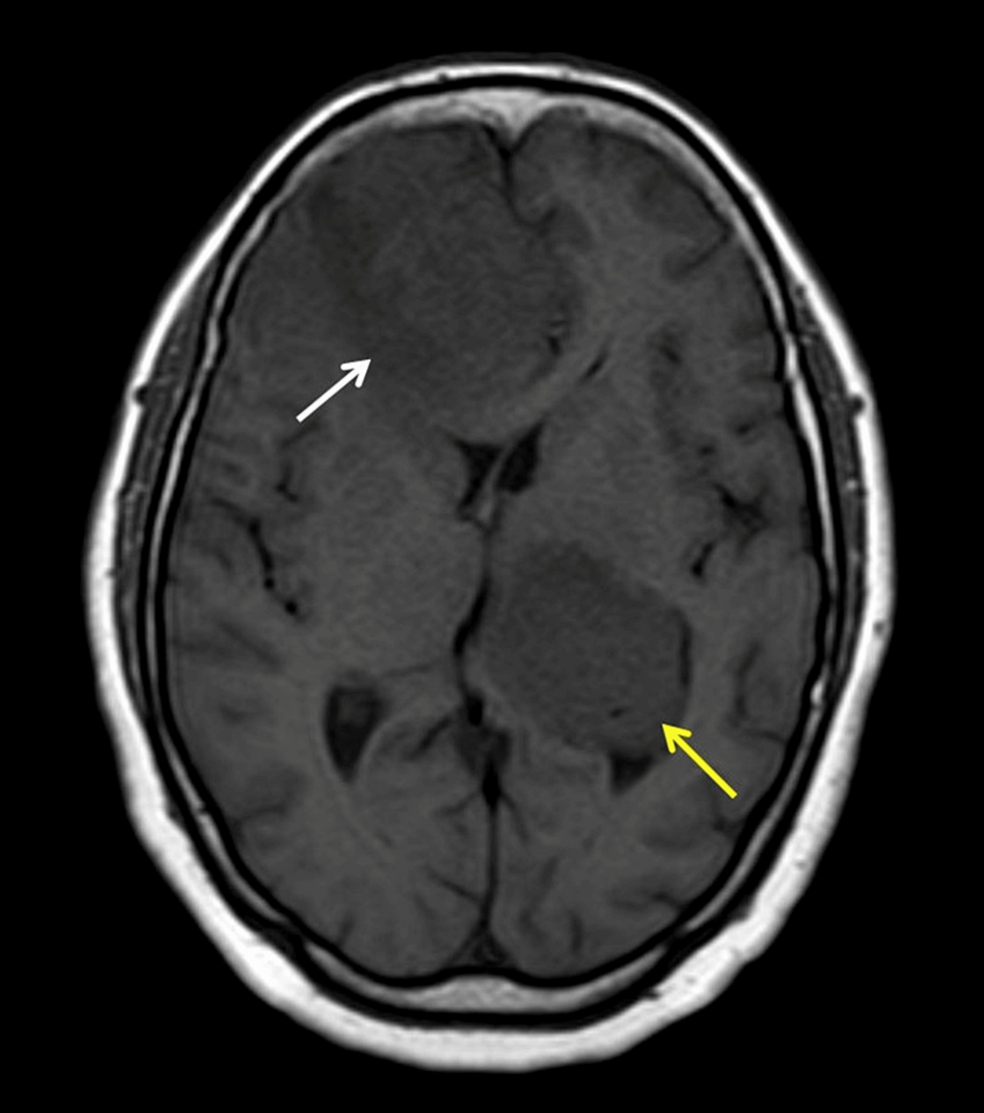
Axial T1 weighted MRI shows coexisting dural meningioma (white arrow) and intraventricular meningioma (yellow arrow)
